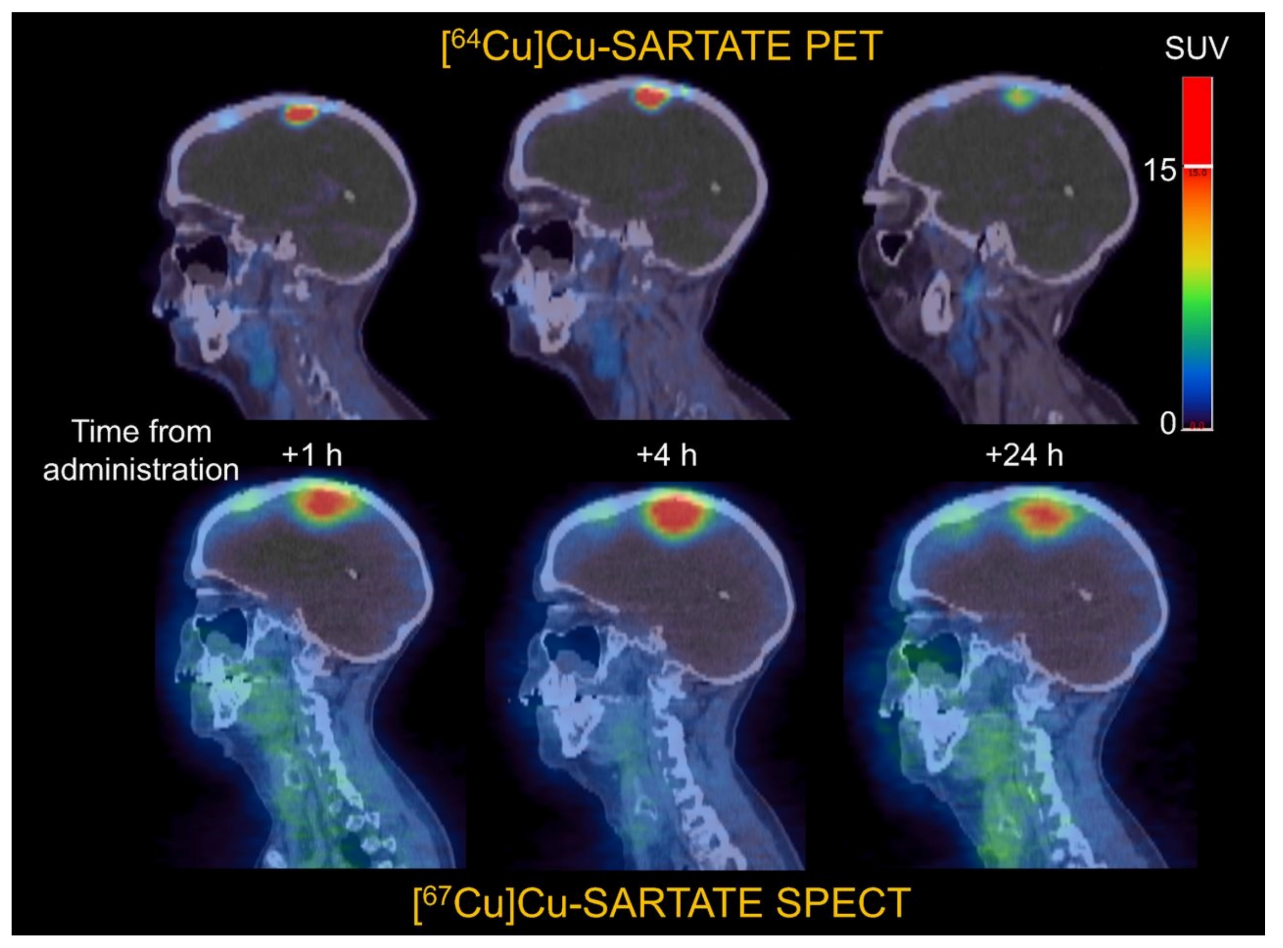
Comparison of clinical ^64^Cu PET imaging and ^67^Cu SPECT imaging, highlighting how changing the radionuclide from ^64^Cu to ^67^Cu does not alter tumor targeting. Adapted with permission from 15, copyright 2022 SNMMI.
